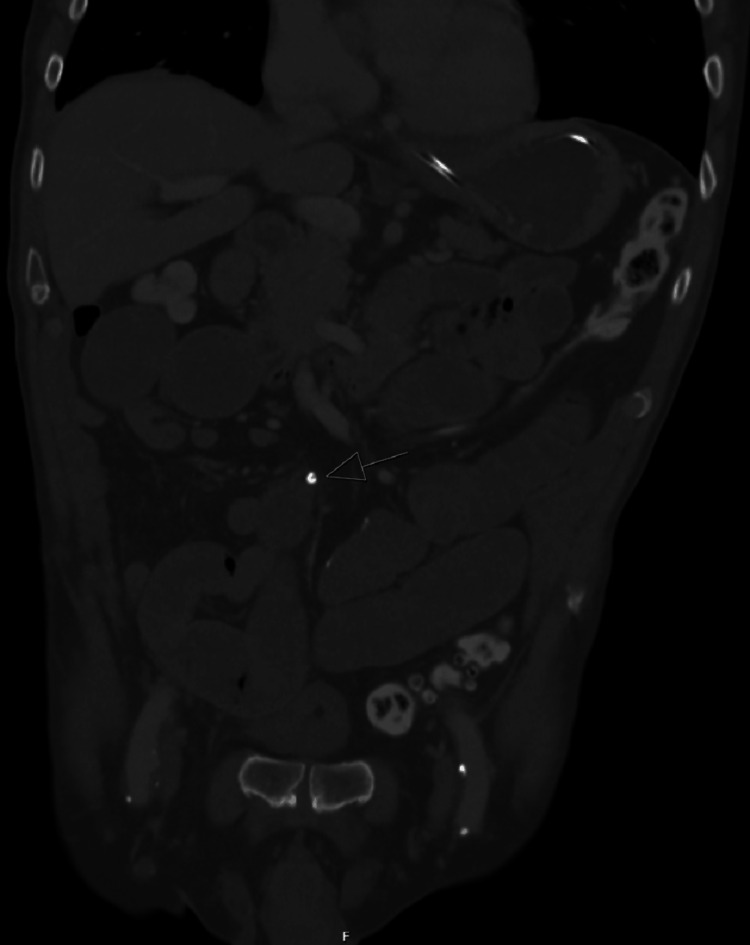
Coronal CT (bone view) image with an arrow showing the loose tack at the transitional point.
